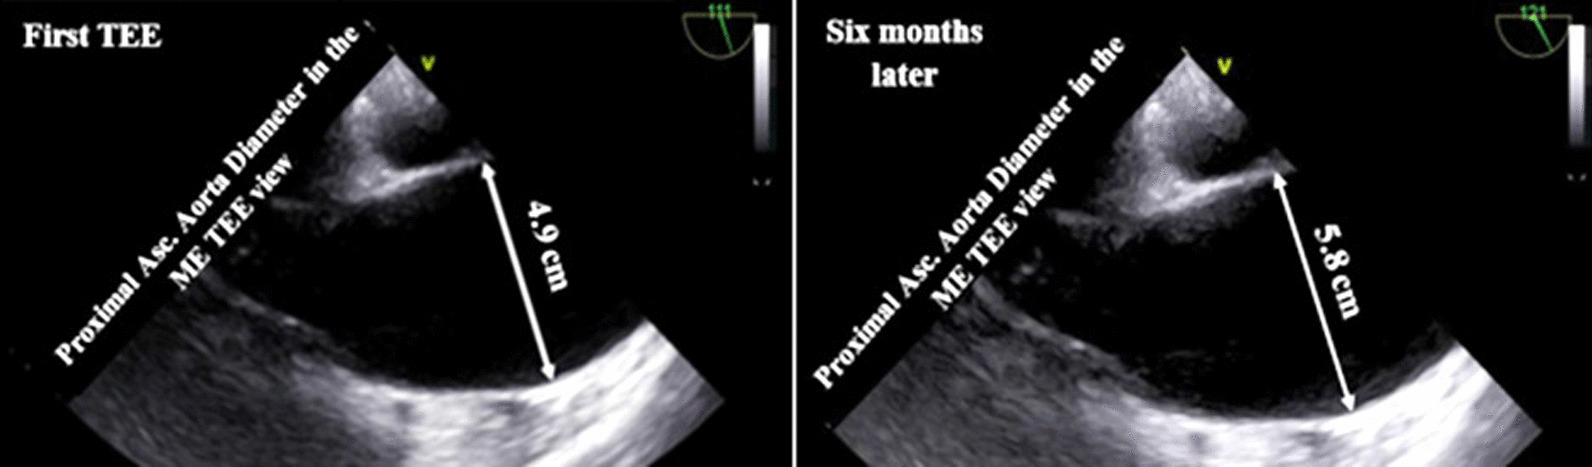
Transesophageal echocardiographic findings of baseline (left) and 6 months later (right); follow-up test showed fast-growing aortic root diameter that reached from 4.9 to 5.8 cm
